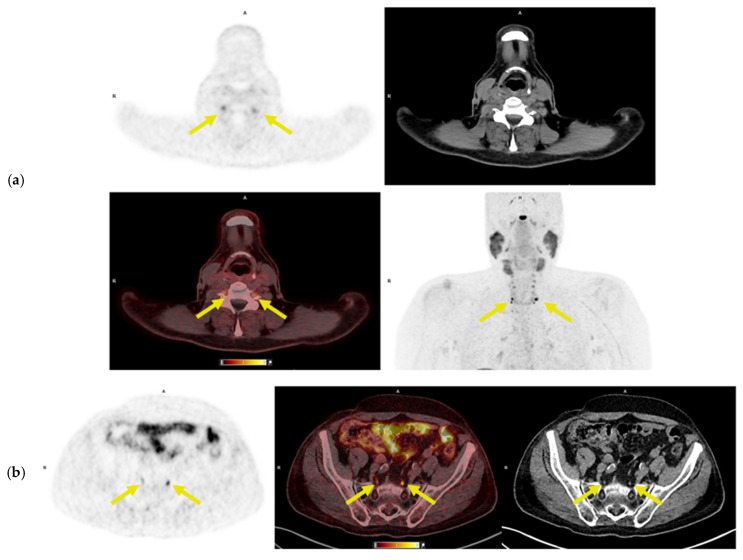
^64^Cu-DOTATATE PET, low-dose CT, fused images (PET/ldCT), and maximum intensity projection (MIP) of the cervical region (a) and pelvic region (b) from a patient with neuroborreliosis. Abbreviations: R = Right, H = Head, A = Anterior. (a): increased ^64^Cu-DOTATATE uptake in cervical and upper thoracic ganglia aligns with upper extremity symptoms of bilateral shoulder pain and finger paresthesia. PET/CT shows uptake (SUV_max_ = 4.6) in the cervical and upper thoracic dorsal root ganglia (yellow arrows). (b): increased ^64^Cu-DOTATATE uptake in the lumbosacral ganglia corresponds to lower extremity symptoms in the same patient as (a). PET/CT shows uptake (SUV_max_ = 5.6) in the lumbosacral ganglia (yellow arrows), matching clinical findings of left leg pain and paresis.
